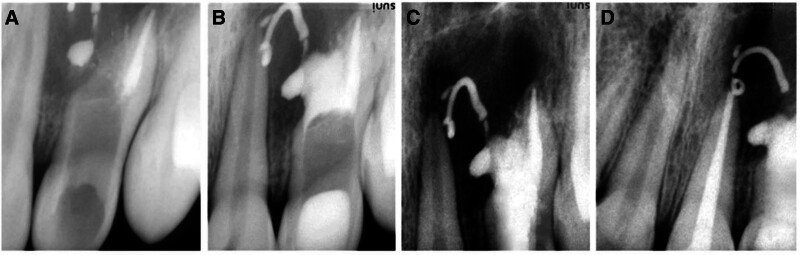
(A) Obturation of regular root canal and removal of intertwining invaginated tissue between the root canal and the pseudo canal in tooth #10. (B) Apexification of the pseudo canal by creating a 4 to 5 mm apical plug using mineral trioxide aggregate in tooth #10. (C) Middle and coronal third of the canal obturated with warm vertical condensation technique using the Obtura III Max in tooth #10. (D) Periapical radiograph of teeth #9, #10 at 6 months recall displaying significant periapical healing.
